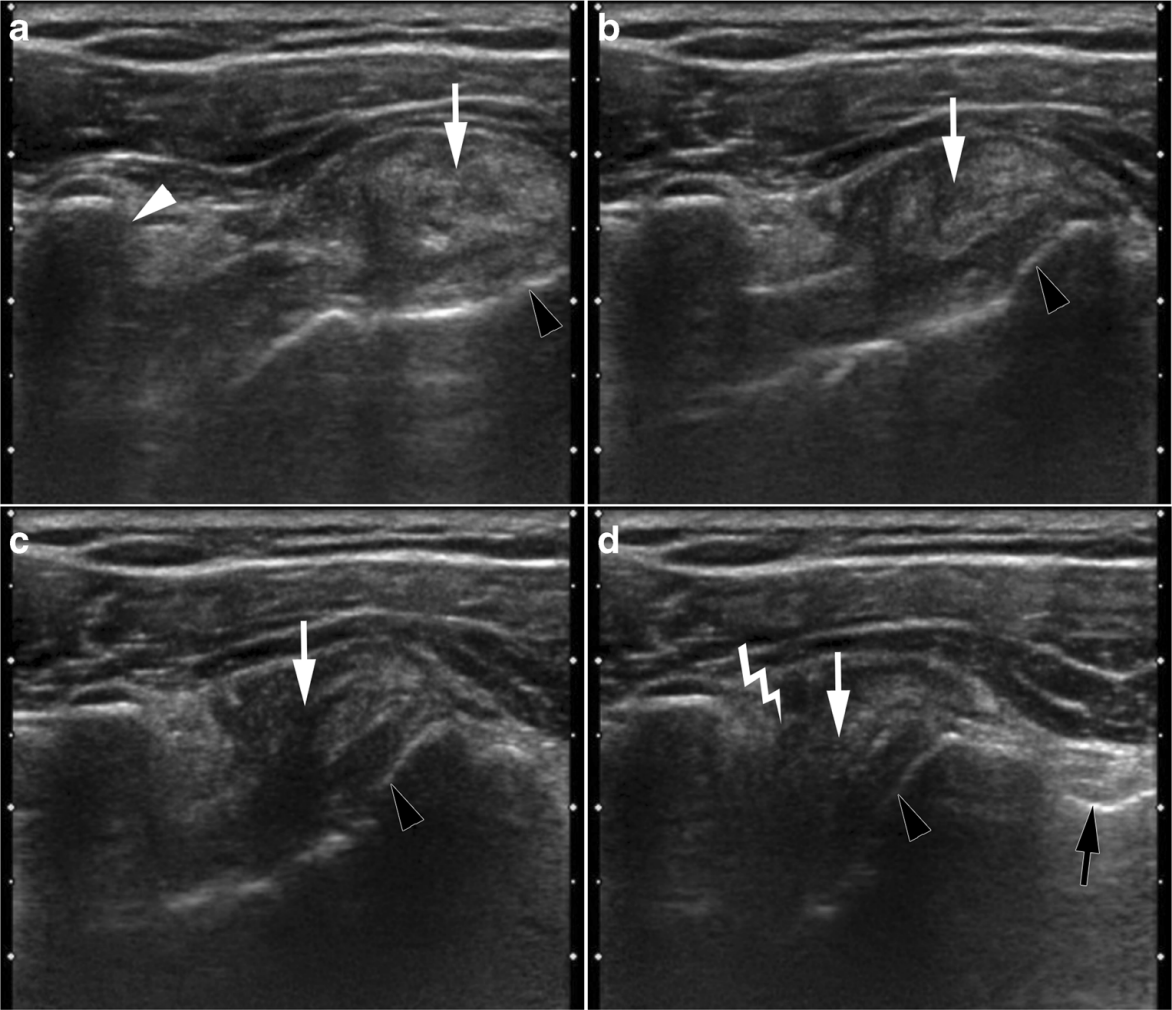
Axial dynamic ultrasound images during progressive internal rotation of the shoulder (from a to d). Anatomic landmarks are the coracoid process (white arrow head), the lesser tuberosity (black arrow heads) and the intertubercular sulcus (black arrow). A macrocalcification is seen in the SSC tendon (arrow). It gradually approaches the coracoid process (b and c). In internal rotation (d), pain and sensation of projection under the probe when calcification passes under the coracoid process, are consistent with coracoid impingement (lightning) associated with calcific tendinitisAn accessory coraco-brachialis muscle is also a constitutional factor of CI, rarely visualized on ultrasound or MRI in front of the SSC [15].
